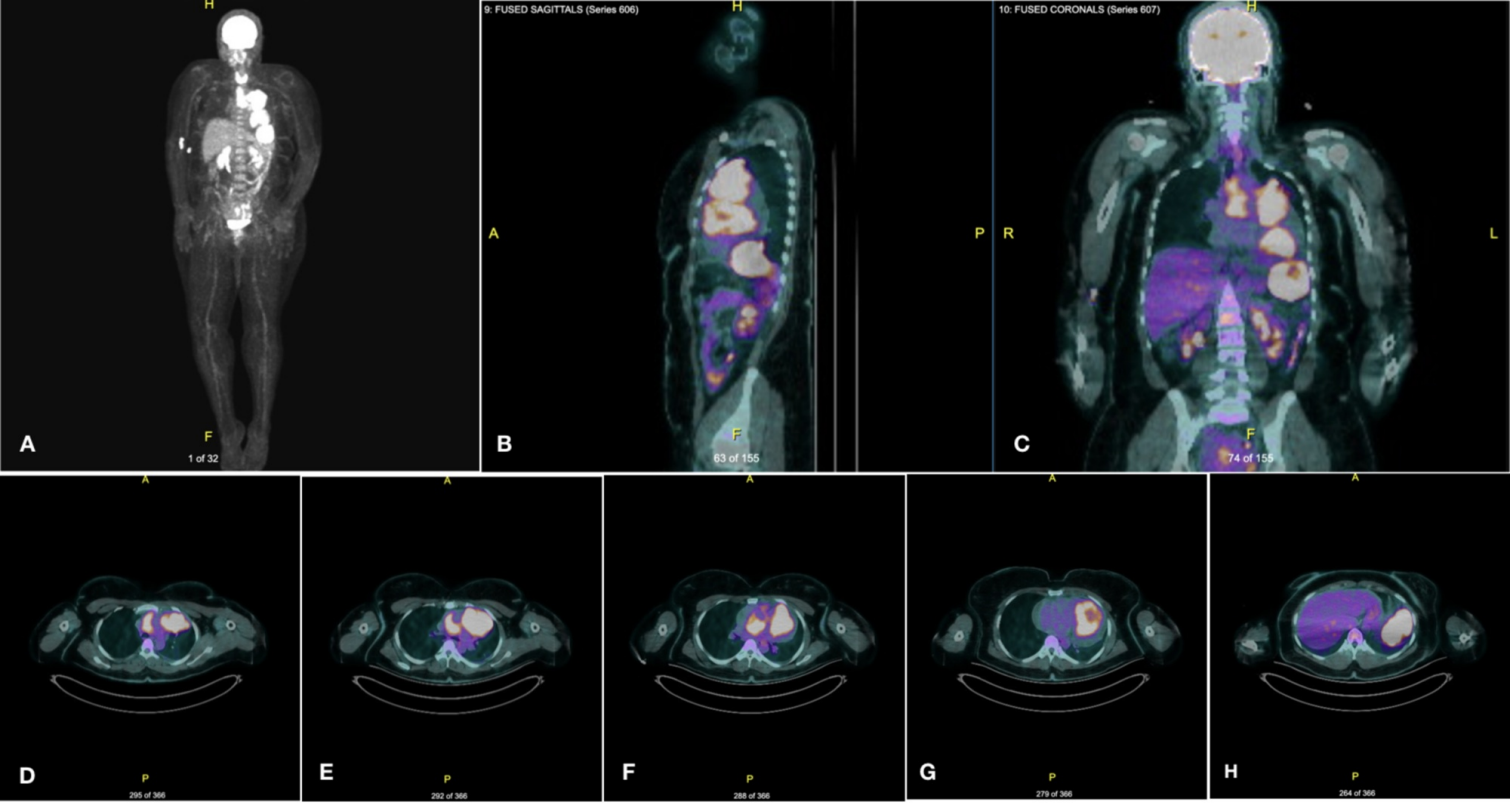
Representative PET/CT scan Maximal intensity projection (MIP) display (A), fused sagittal view (B), fused coronal view (C), and fused horizontal tomographic renditions (D-H) demonstrate intrathoracic neoplastic burden, highlighting the tumor’s close proximity and direct invasion of cardiac and vascular structures PET/CT: positron emission tomography-computed tomography
